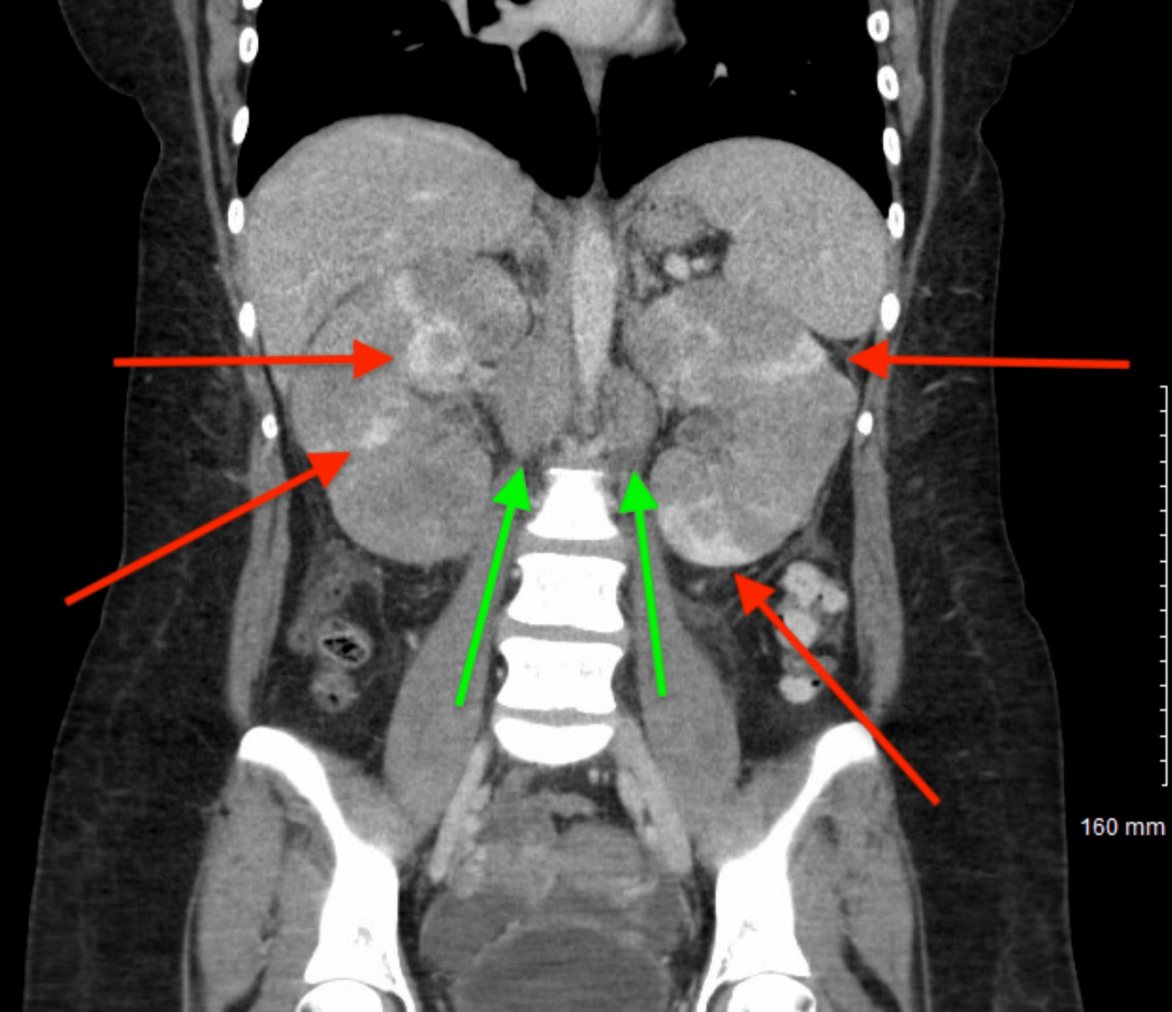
Contrast-enhanced CT abdomen and pelvis demonstrating renal cortical lesions and retroperitoneal lymphadenopathy Contrast-enhanced CT showing multifocal bilateral renal cortical hypoenhancing lesions with similar distribution compared with prior imaging (red arrows). There is also interval progression in the size and conspicuity of retroperitoneal lymph nodes, most prominent in the periaortic and aortocaval regions (green arrows), concerning for progression of an underlying lymphoproliferative process.
